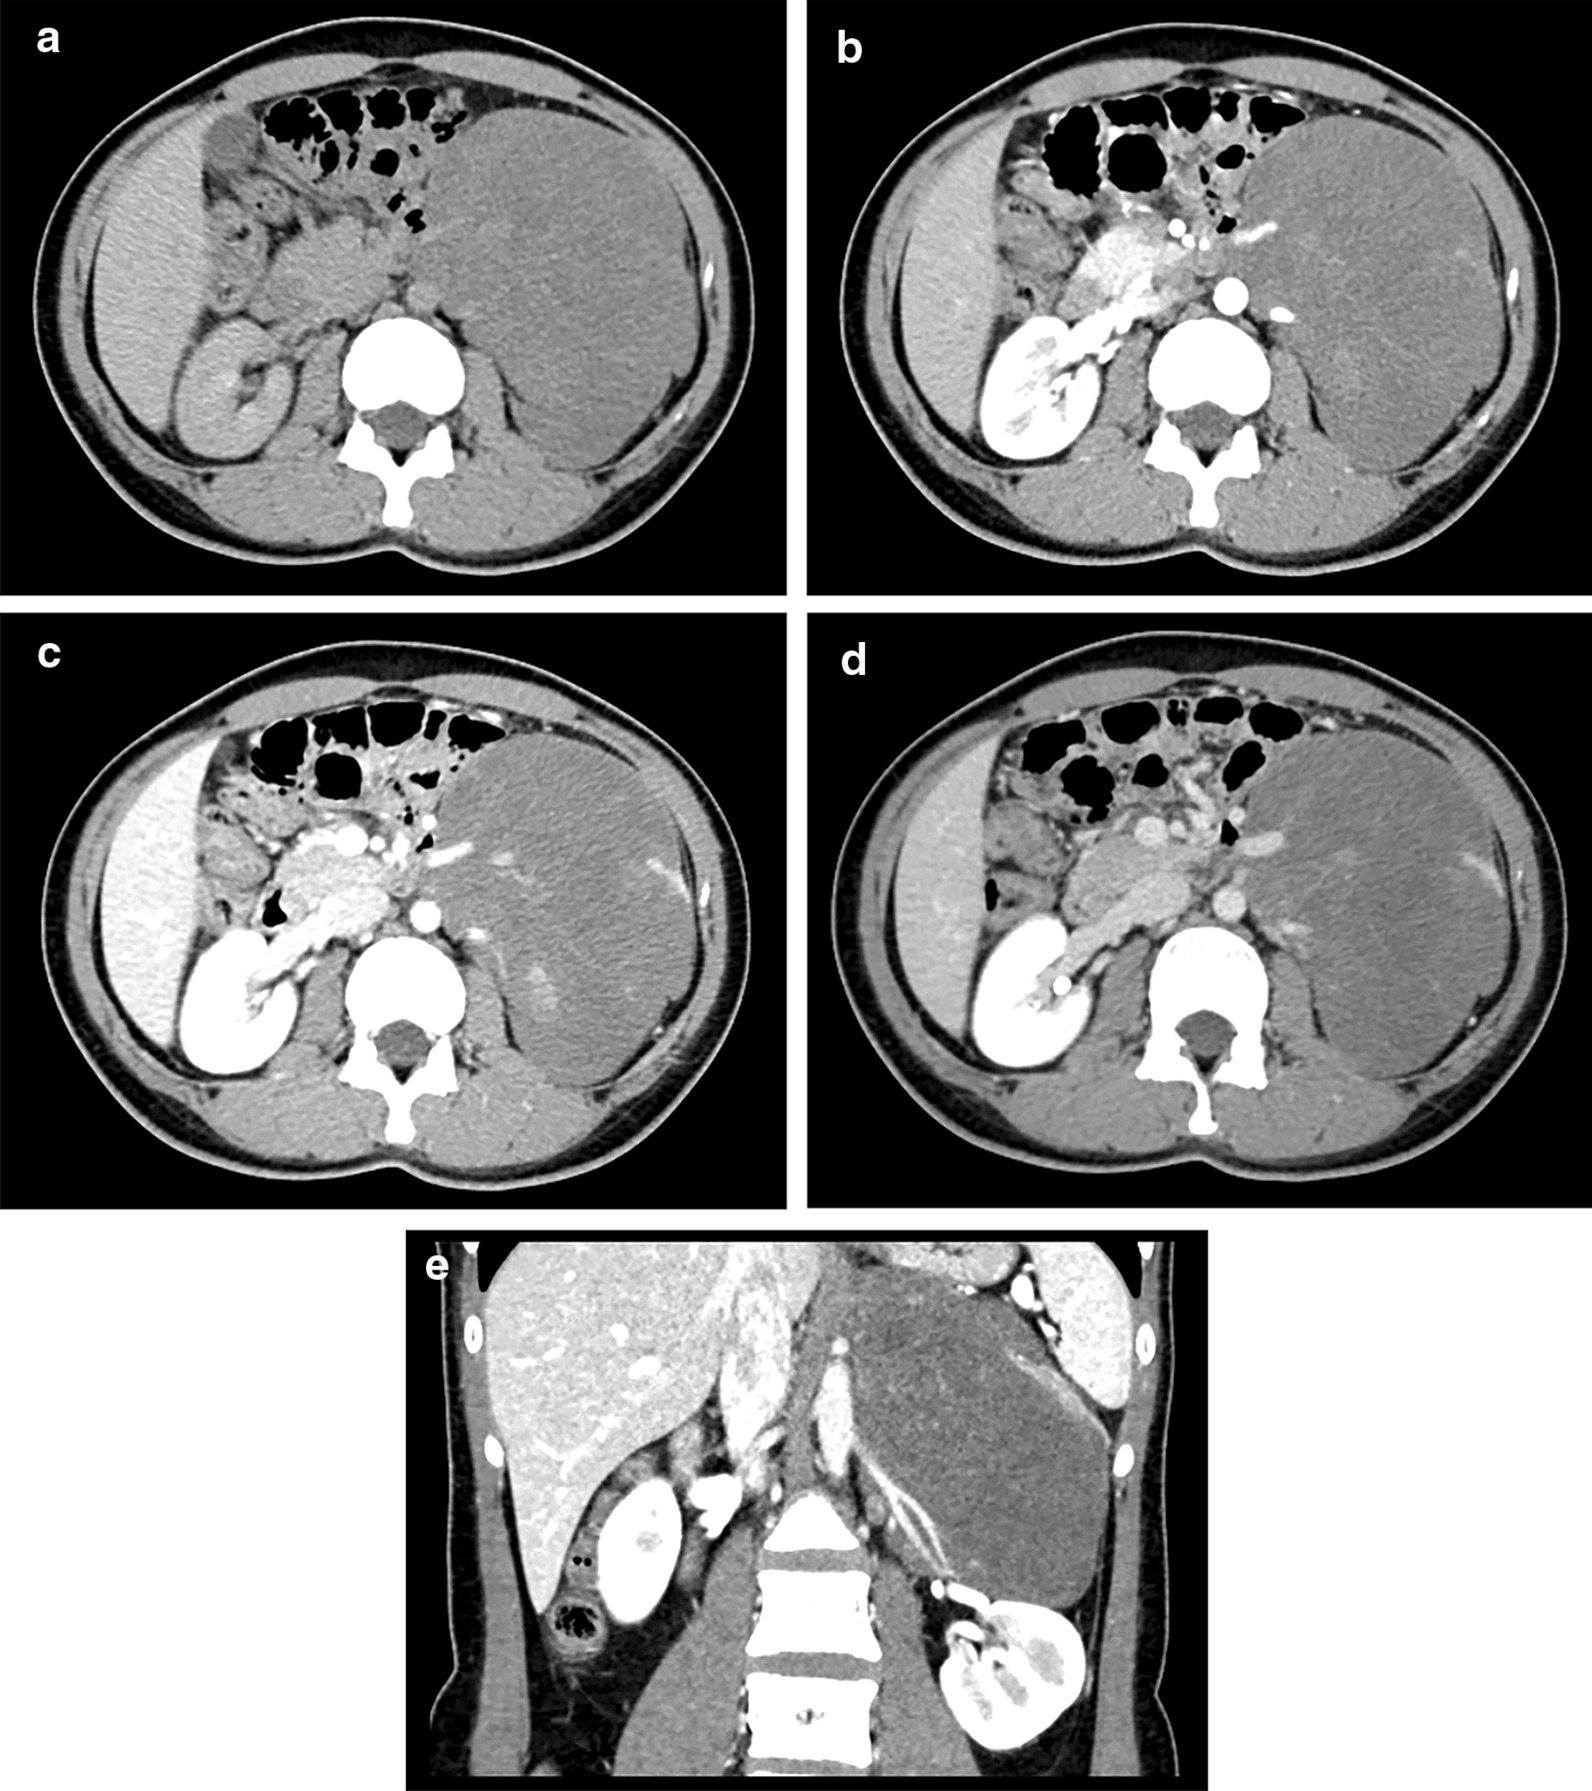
CT image of a 22-years-old female (patient no.3). The retroperitoneum ganglioneuroma was incidentally detected by the health examination after college graduation. a Non-enhanced image. b arterial phase. c portal phase. d delayed phase. e coronal image. The tumor encased the left renal pedicle, and the patient lost 900 ml blood during surgery
